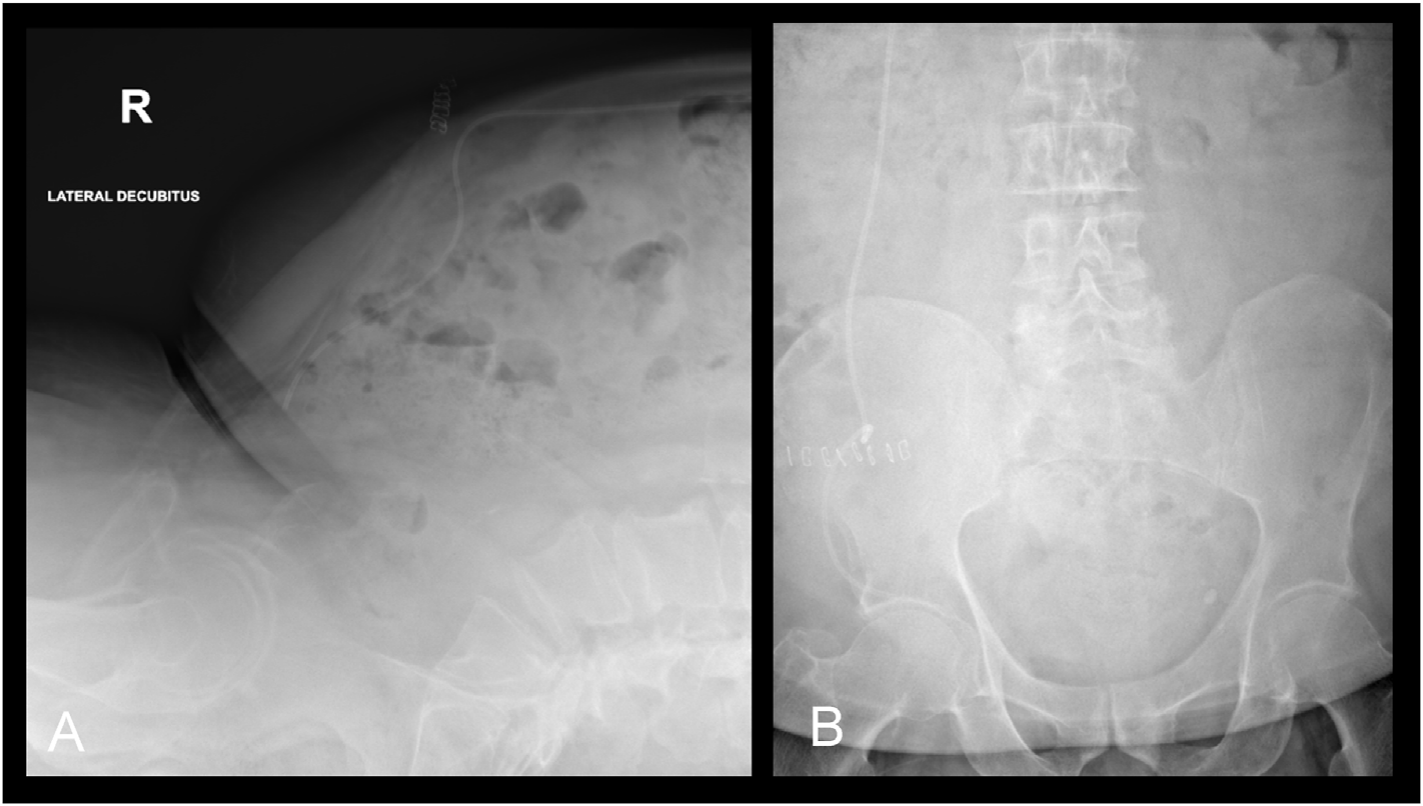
Postoperative radiographs in lateral (A) and anteroposterior (B) projections showing truncation and adequate placement of the distal shunt catheter.
